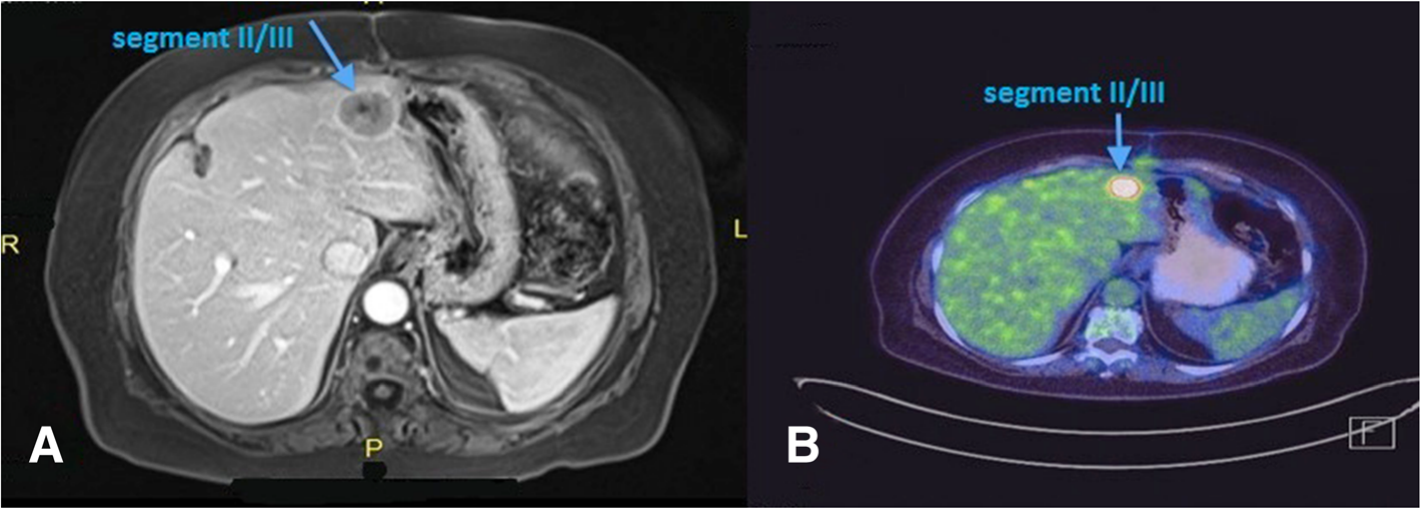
a MRI (4 months post-liver resection): focal lesion at segment II/III of the liver measuring 2.5 cm in diameter, with signal intensity and enhancement pattern impressive of metastasis. b PET scan 4 months post-liver resection: intense uptake in the left lateral aspect of the left liver lobe (segment II/III) corresponding to an approximately 2 cm sized hypodense lesion. New intense uptake corresponding to hypodense lesion in the left liver lobe consistent with new liver metastasis
